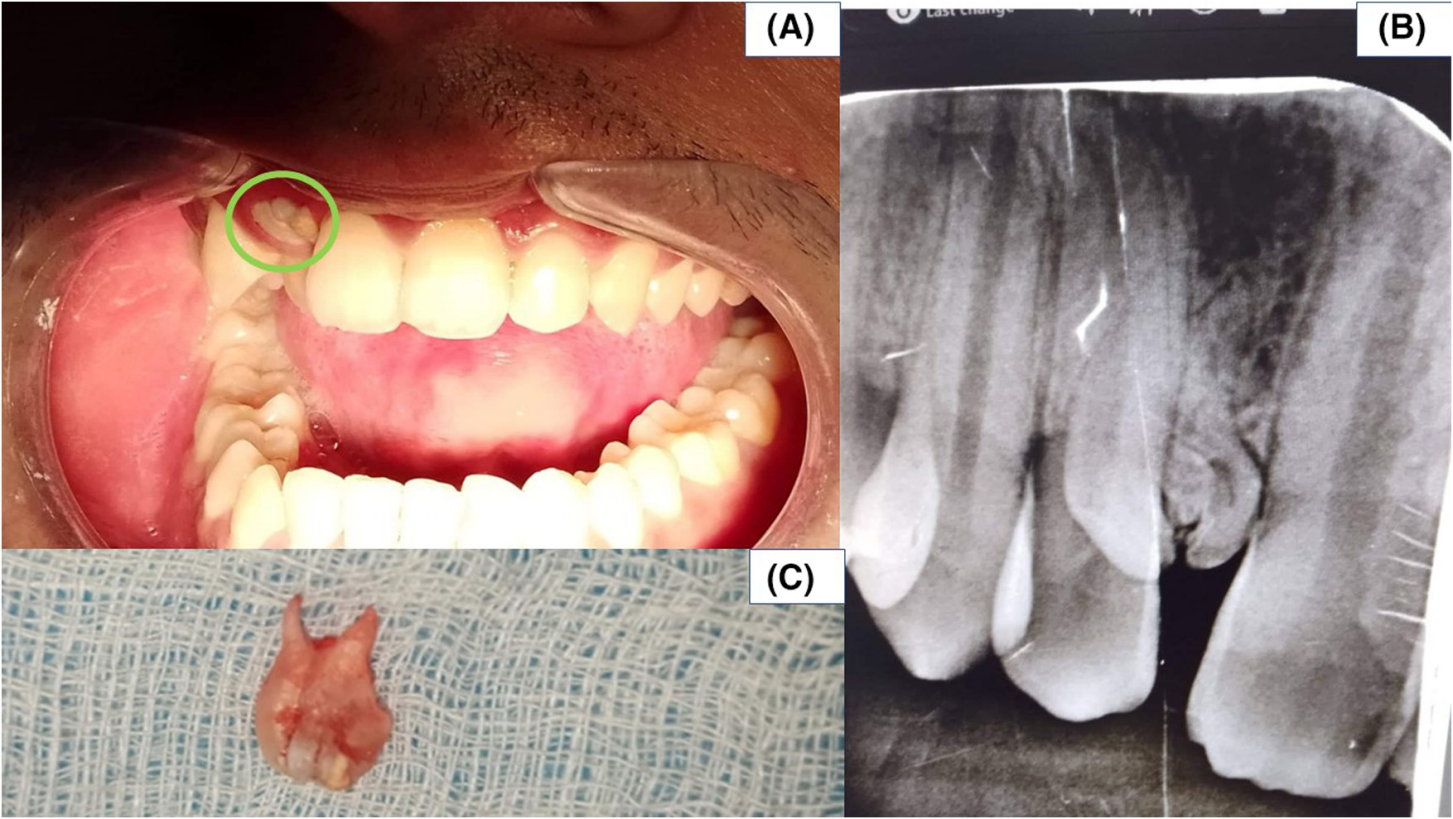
(A) Diffuse white mass, protruding from the maxillary gingiva, adjacent to the right maxillary central incisor. (B) Intra‐oral periapical radiograph showed a tooth‐like mass. (C) Excised mass showed a multirooted tooth partially resembling a primary molar
